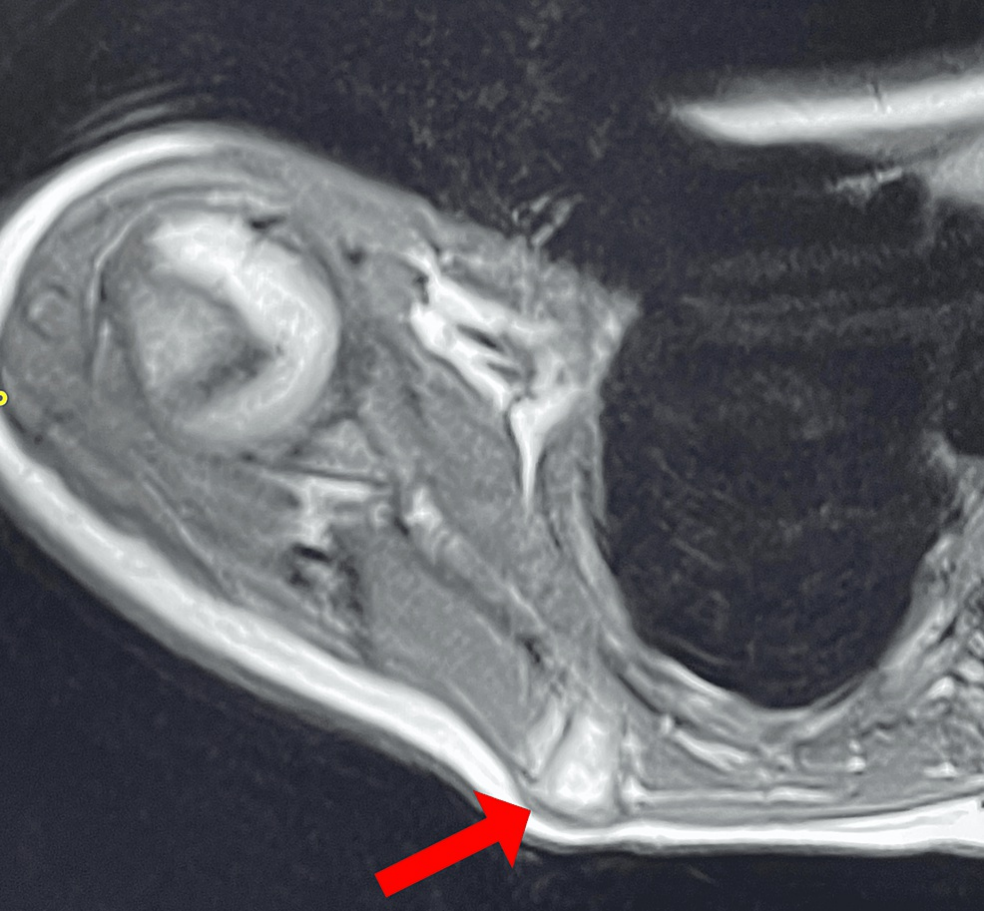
MRI showing abnormal bone growth. The image shows an exostosis of the right scapula (arrow).
